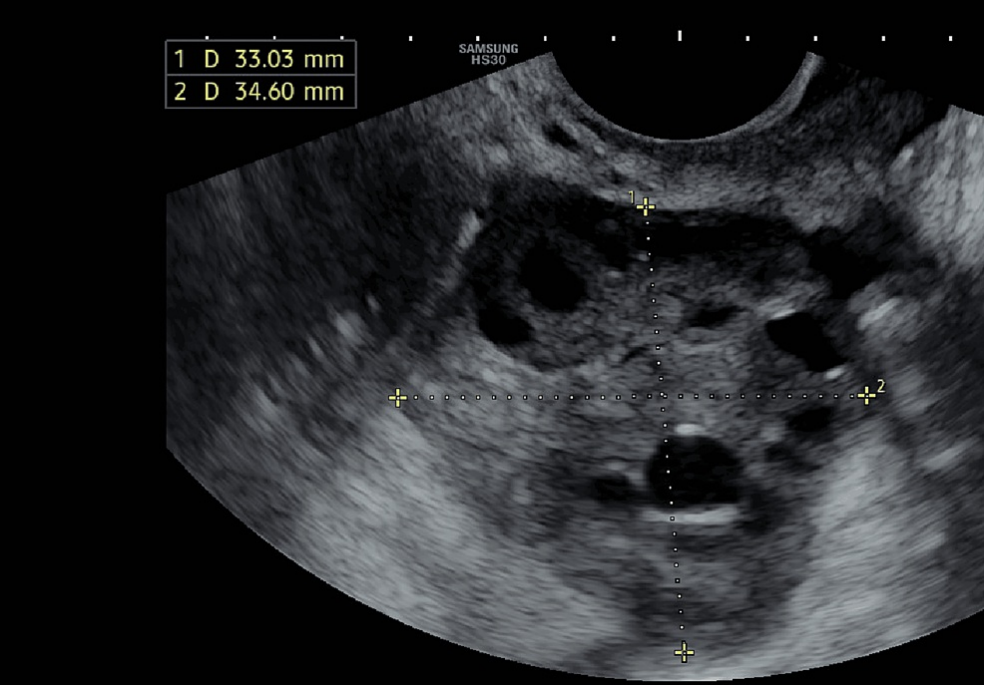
Transvaginal sonography image of the patient's right ovary on day two of the menstrual cycle
